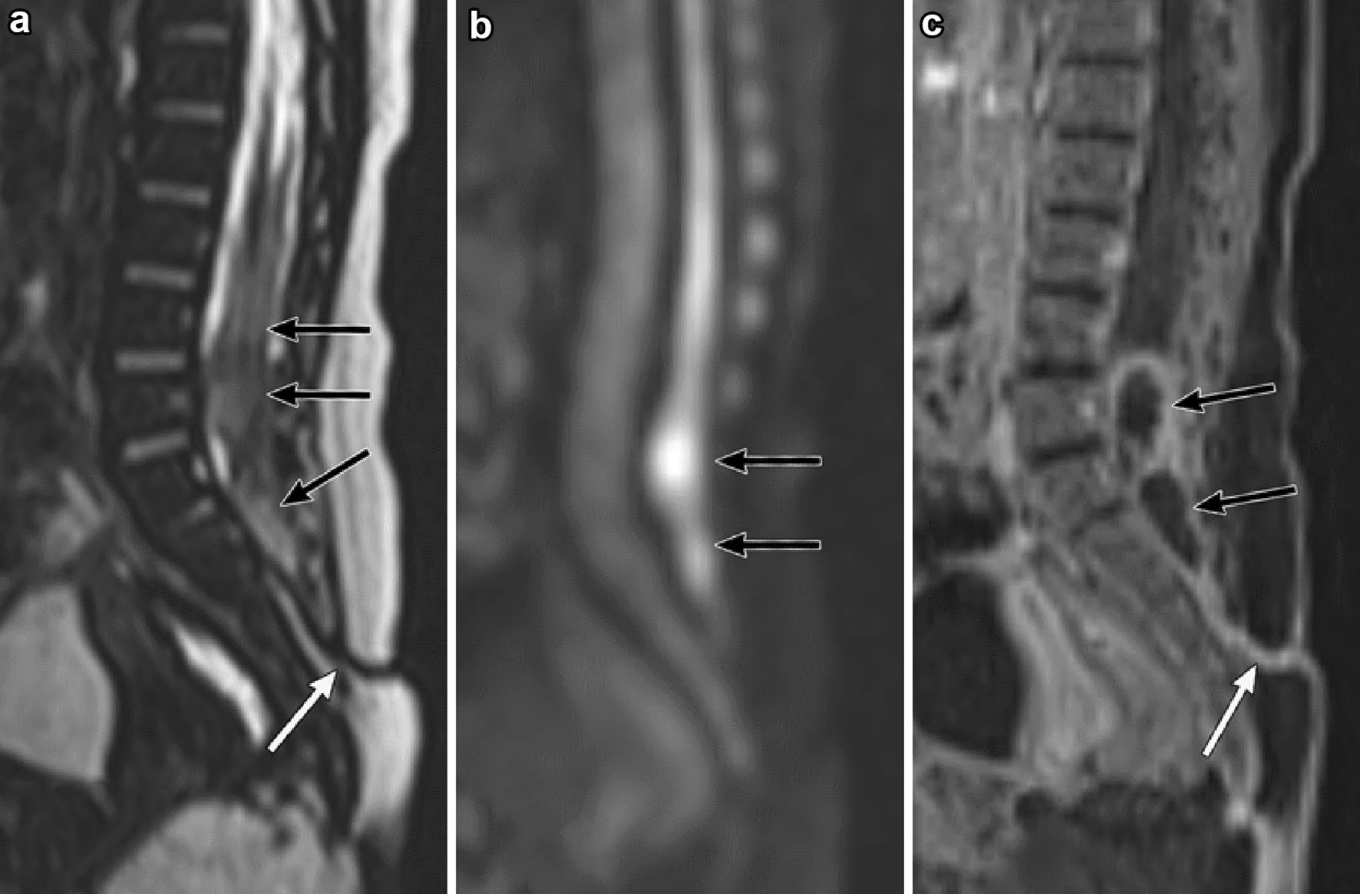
A spinal dermal sinus tract (DST) in a 3-month-old girl with bacterial meningitis (BM). A sacral dimple was first noted during her hospitalization for BM. a Sagittal T2-weighted magnetic resonance (MR) image shows a low conus medullaris. The caudal end of the spinal cord is tethered by a DST (white arrow). The spinal cord is thickened, and hyperintense tumor-like structures are observed in the dural sac (black arrows). b Diffusion-weighted image shows the tumor-like structures with diffusion restriction in the dural sac (black arrows). c Gadolinium-enhanced fat-suppressed T1-weighted MR image shows that the tract (white arrow) and rim of the tumor-like structures (black arrows) are enhanced. The differential diagnoses at this point include an infected dermoid or epidermoid and intraspinal abscess in the dural sac. Surgical resection of the DST and untethering of the spinal cord were performed. An intraspinal abscess and a dermoid tumor in the dural sac were also resected during surgery
